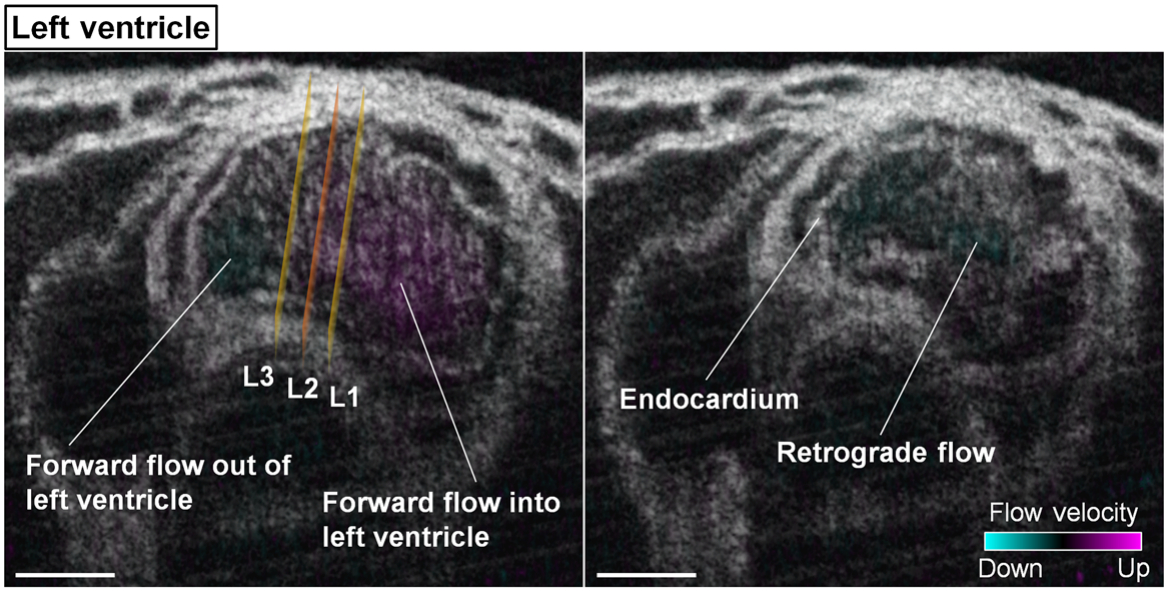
Structural and Doppler 4D-OCT imaging of the left ventricle of E9.25 embryonic heart (Video 3). The positions of the measurement planes L1, L2, and L3 are shown in the left panel. Scale bars are 100  μm (Video 3, mov, 9.96 MB [URL: https://doi.org/10.1117/1.JBO.25.8.086001.3]).
